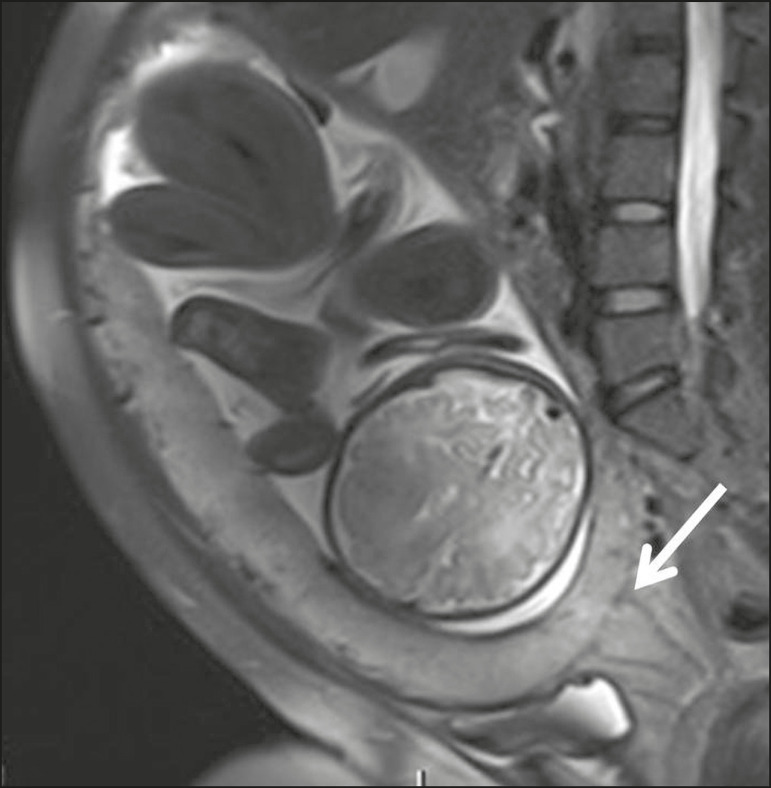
Sagittal T2-weighted MRI sequence showing placental protrusion into the internal cervical os.
